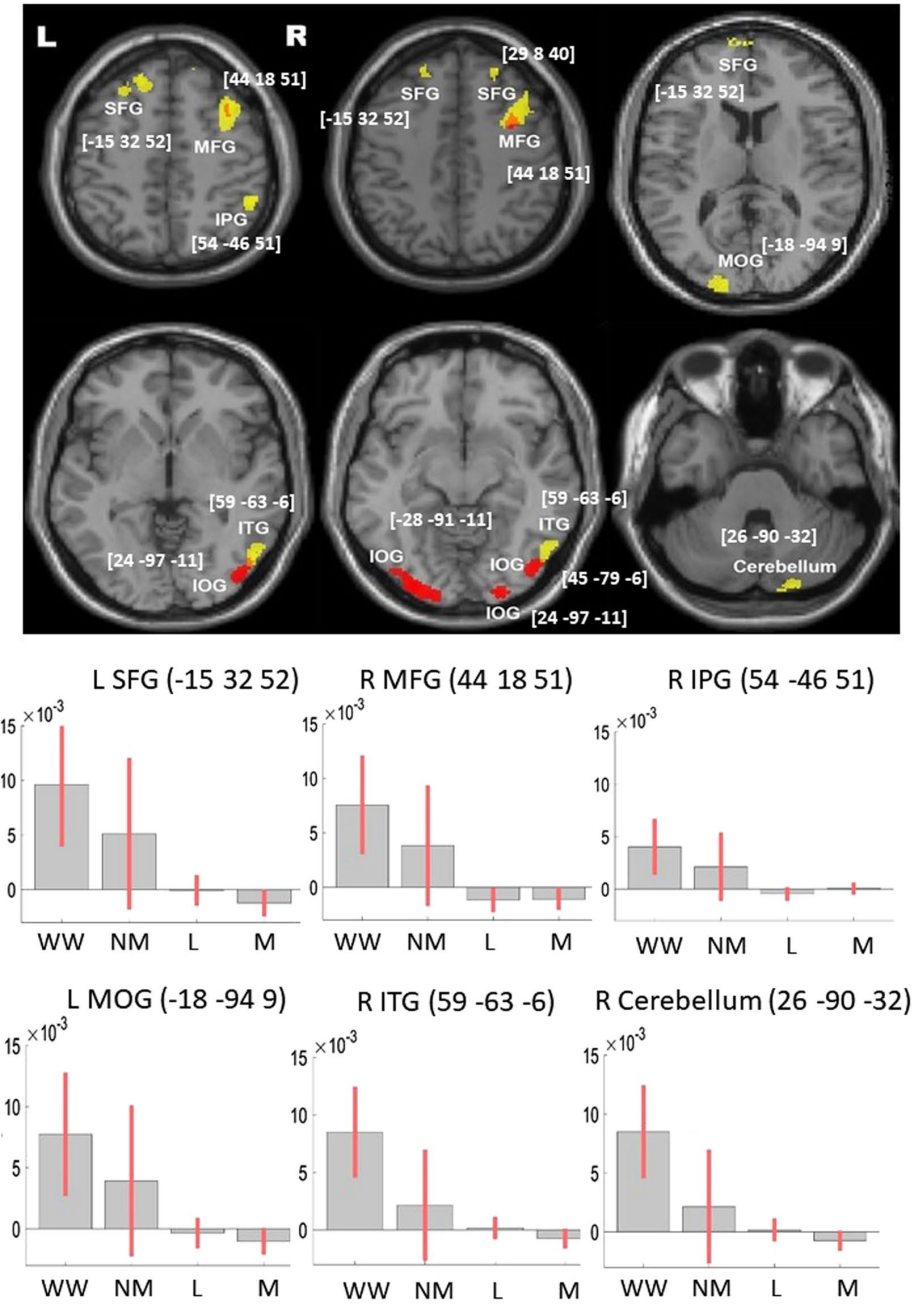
VBM results with raw scores (model 1 & 2) (N = 267). The VBM results overlaid on the canonical T1 images (SPM), showing lesions associated with deficits in both writing tasks (conjunction). Results of model 1 (model not included other language and motor tasks) are presented in red, and for model 2 (model included the 4 language and 3 motor tasks) in yellow. The charts represent effect size (beta) for each writing tasks and the average effect size for the language (L) and motor (M) tasks. The error bars are 90% confidence interval of the effect size. Abbreviations: L, left, R: right, SFG, superior frontal gyrus; MFG, middle frontal gyrus; IPG, inferior parietal gyrus; MOG, middle occipital gyrus; ITG, inferior temporal gyrus, IOG, inferior occipital gyrus; WW, word writing; NW, number writing; L, language, M, motor. See Supplementary Figs. 2 and 3 for case examples of lesion on the CT scans.
